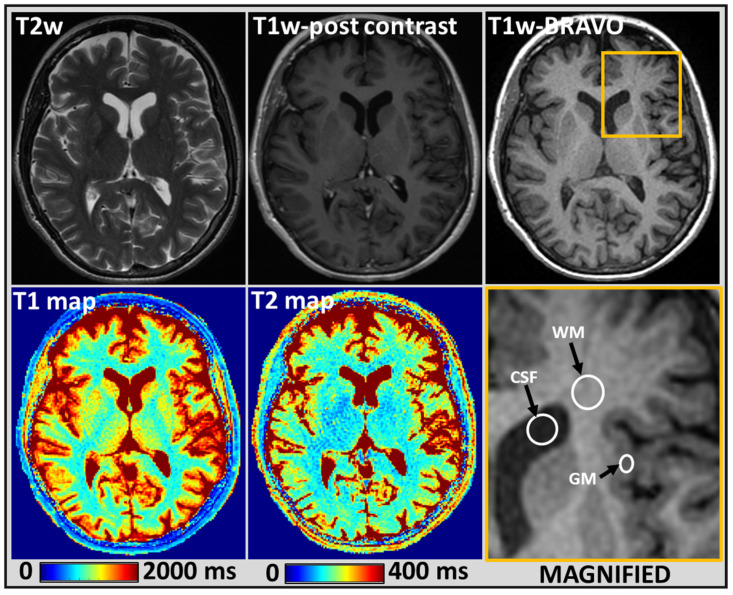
Representative MR images showing normal-appearing brain tissue from a 52-year-old female patient treated with focal RT and had a history of primary melanoma: Conventional T2-weighted (w), post-contrast T1w and BRAin VOlume (BRAVO) images depicting the anatomical structures (top row). T1 and T2 maps estimated using MRF for a single slice exhibiting gray matter (GM), white matter (WM), and cerebrospinal fluid (CSF) (bottom row). The yellow outlined region on a BRAVO image was magnified, and the placement of region of interest (ROI) is in white for normal-appearing GM, WM, and CSF. The MRF estimated mean T1 values for these normal-appearing GM, WM, and CSF regions were 1204 ms, 842 ms, and 4190 ms, respectively, and the mean T2 values were 108 ms, 78 ms, and 442 ms.
